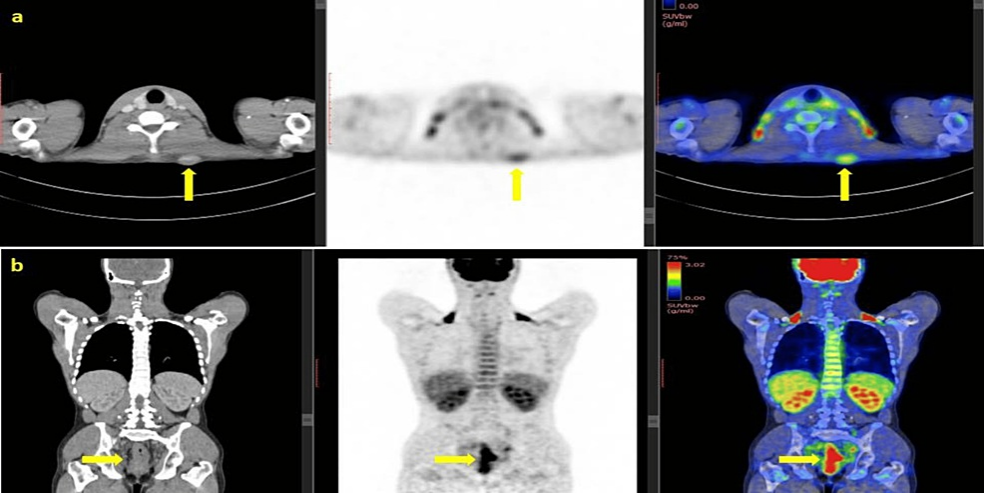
FDG-18 PET-CT scan demonstrated hypermetabolic lesion (a) at the nape of neck and (b) primary hypermetabolic rectal tumor (yellow arrows).
